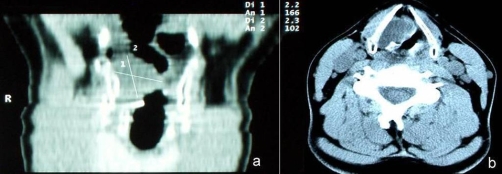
Preoperatively (a) coronal and (b) axial CT sections of the larynx to the level of the true vocal cords showed an exophytic tumour, which occupied the largest part of the glottis extending from the right arytenoid region to the anterior third of the left vocal cord.
